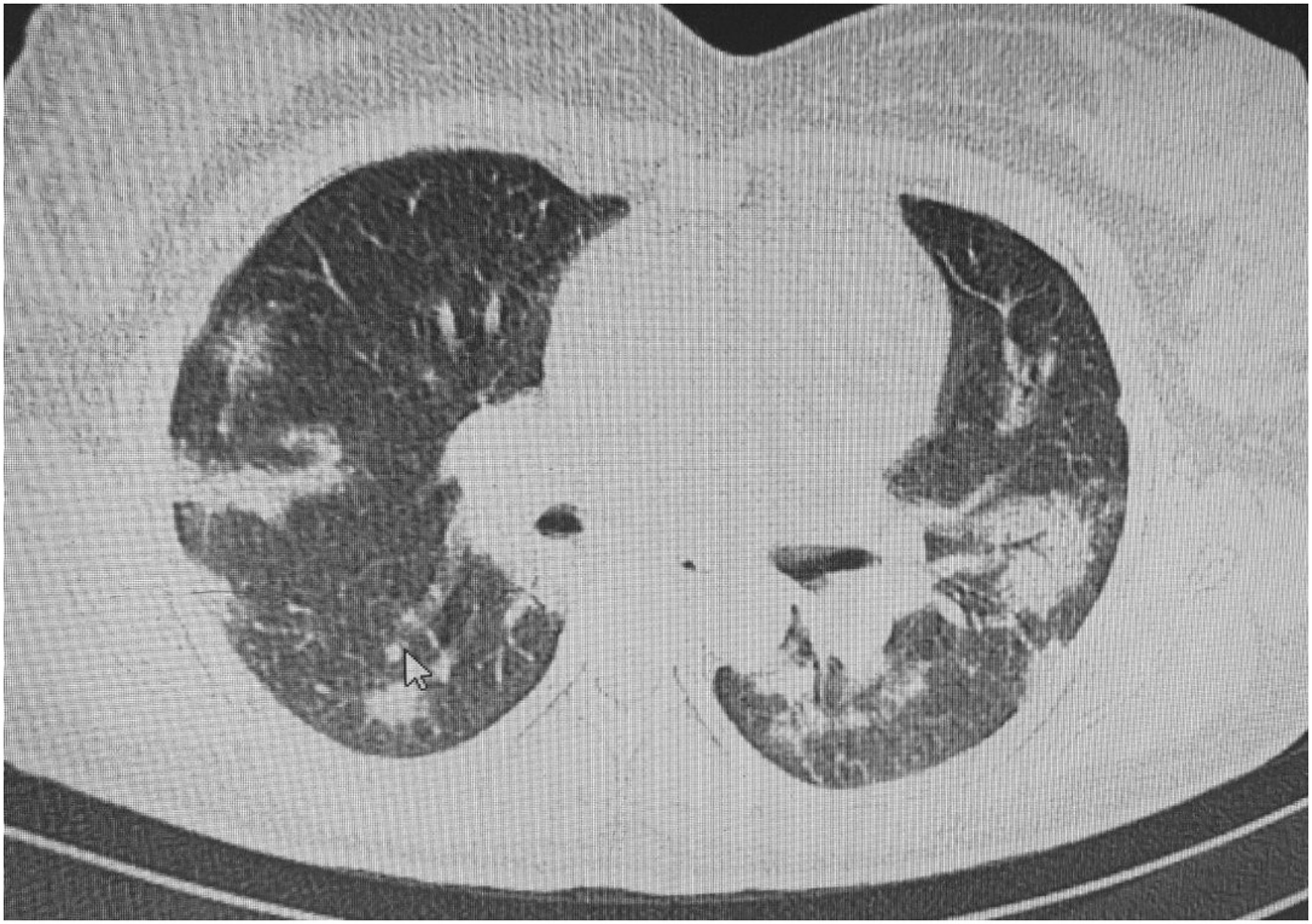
High resolution chest CT‐scan of the patient with pulmonary involvement following Covid‐19 infection showing bilateral patchy infiltration with subpleural involvement in some areas and mild grand glass opacity.
